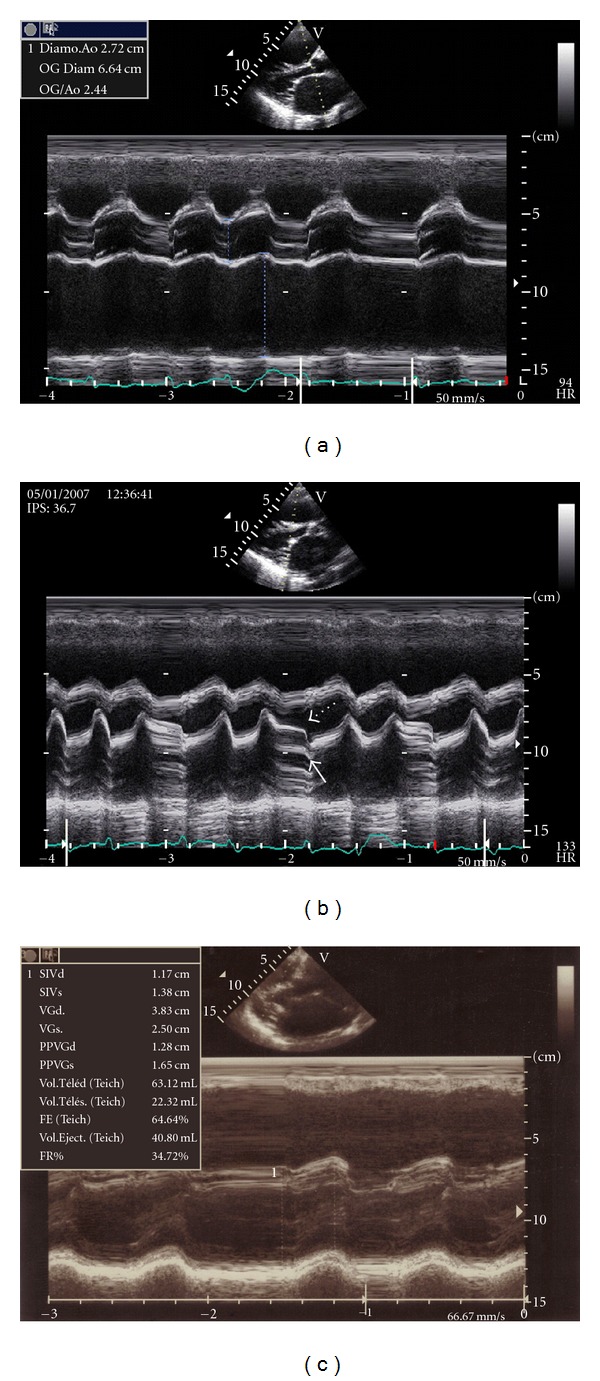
Transthoracic echocardiography, left parasternal long axis view M-mode. (a) dilatation of the left atrium. (b) Paradoxical anterior motion of posterior mitral leaflet (white arrow), with reduced separation of the two leaflets. Reduction of EF slope (broken arrow). (c) Normal left ventricular size, paradoxical septal motion.
